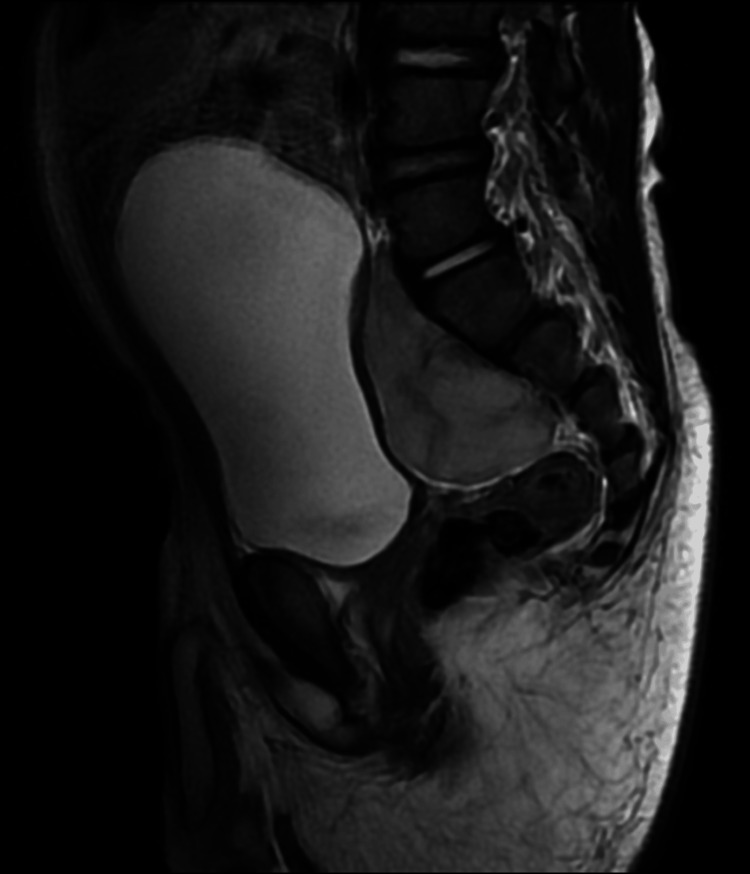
A sagittal image of MRI showed a solid mass on the right side of the pelvis posterior to the urinary bladder and was seen extending to the right sacral foramina.
